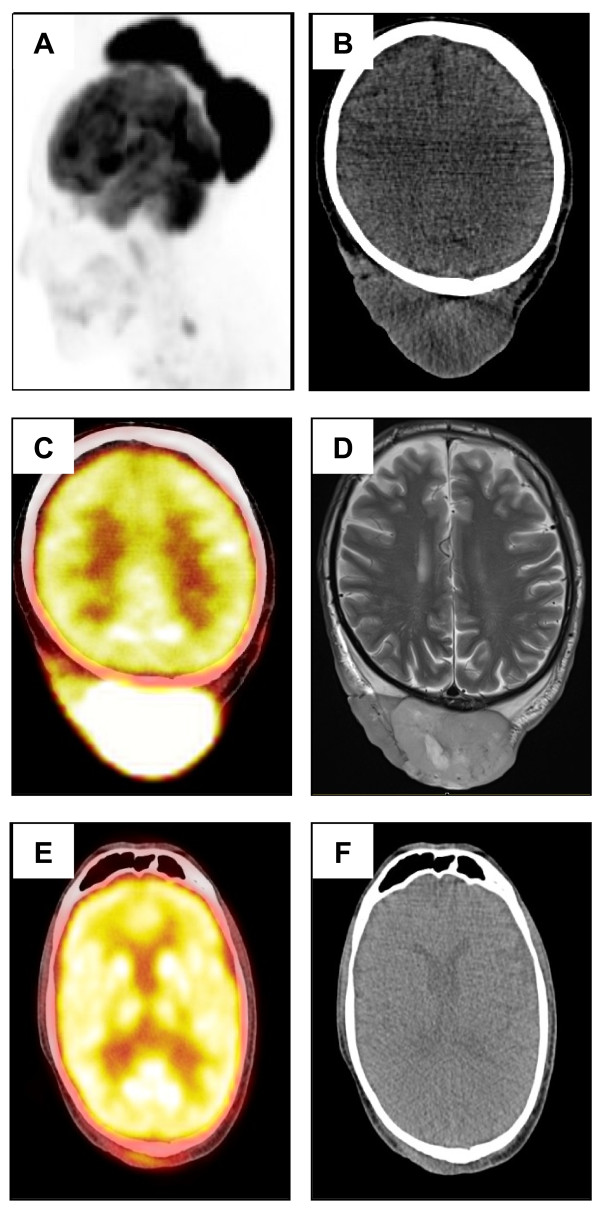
Radiological investigations showing the lesions before treatment and their evolution after the treatment. MRI at diagnosis (A), (B); PET-CT at diagnosis (C), (D); PET-CT and MRI after 2 cycles of R-CHOP (E), (F).
